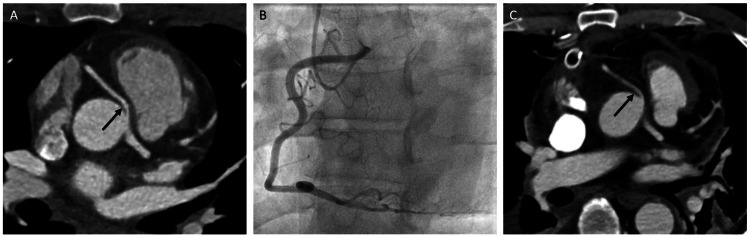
Imaging findings Axial contrasted CT scan (A) of the chest showing an anomalous RCA arising from the left sinus of Valsalva coursing between the aorta and right ventricular outflow tract. Coronary angiography (B) showing the RCA with an anomalous takeoff from the left coronary cusp. Post-operative axial contrasted CT scan (C) of the chest showing a widely patent RCA
